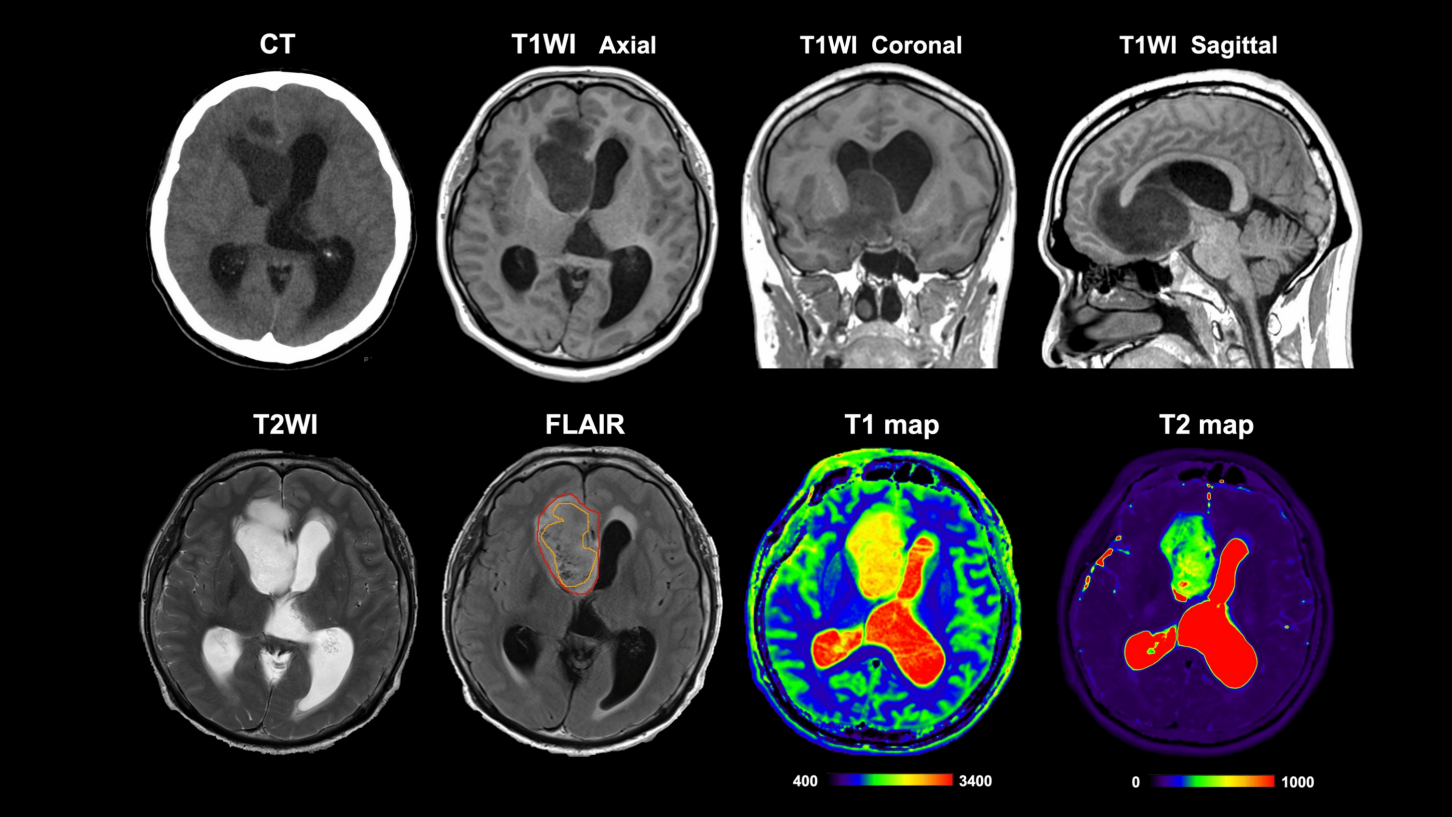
Radiological images before treatment A space-occupying lesion within the right lateral ventricle is observed on computed tomography (CT) and magnetic resonance imaging (MRI) axial images. The sagittal section of the T1-weighted image (T1WI) suggests that the tumor has arisen from the subcallosal gyrus, extending to the anterior cingulate gyrus and putamen. T2-weighted imaging (T2WI) and fluid-attenuated inversion recovery (FLAIR) sequences show a typical "T2-FLAIR mismatch sign." Quantitative magnetic resonance (MR) relaxometry suggests the tumor to be an astrocytoma, isocitrate dehydrogenase (IDH)-mutant. The region of interest (ROI) crafted on FLAIR was placed at the tumor lesion on both T1 and T2 images. The red ROI indicates the entire lesion, while the orange ROI indicates the T2-FLAIR mismatch region.
